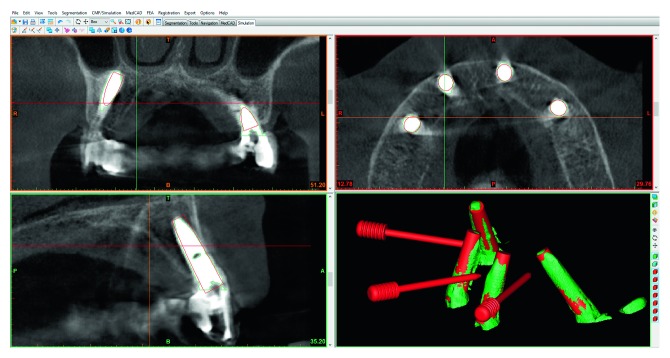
Super imposition of the postop CT scan and the preop 3D panning.
